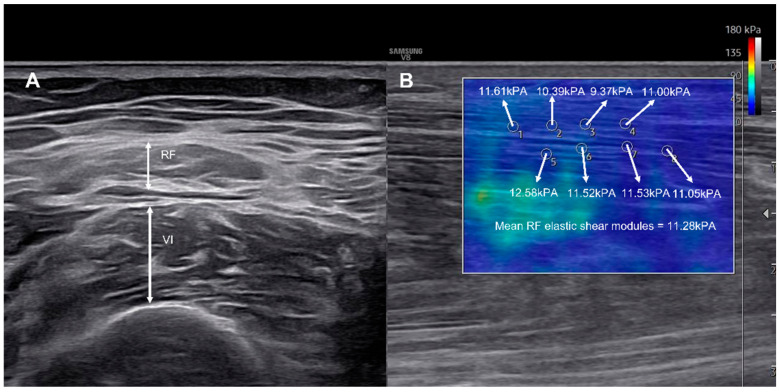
Representative ultrasonographic images showing measurement of RF and VI muscle thickness (A), and corresponding shear-wave elastography map (B). The color scale indicates the elastic shear modulus (kPa) ranging from 0 to 180 where blue represents softer tissue and red indicates stiffer tissue. Multiple regions of interest (ROIs) were positioned along the muscle fascicles, and mean shear modulus was automatically calculated by the system.
